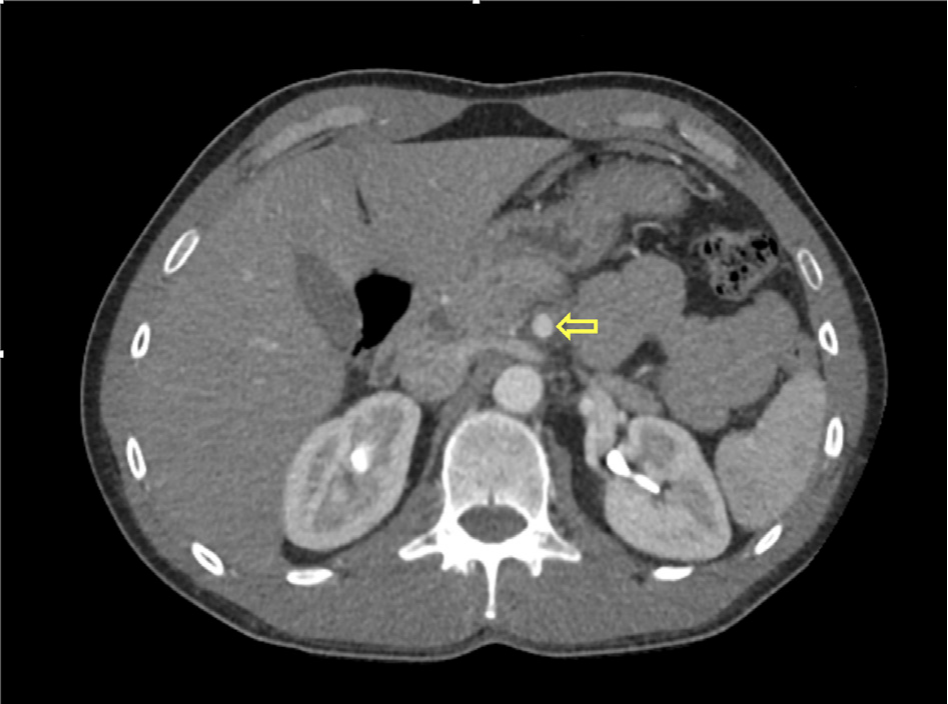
The 3-month follow-up computed tomography angiography image of the abdomen revealing remodeling of superior mesenteric artery and resolution of the dissection.
